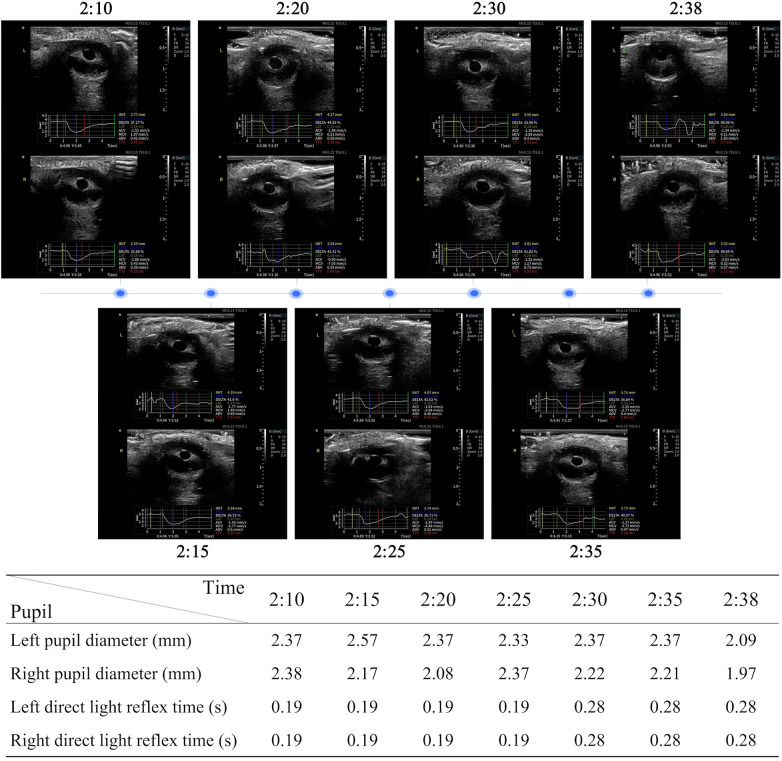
Preoperative pupil images of patients obtained using an automated ultrasonic pupil assessment device to determine pupil diameter and light reaction time.
